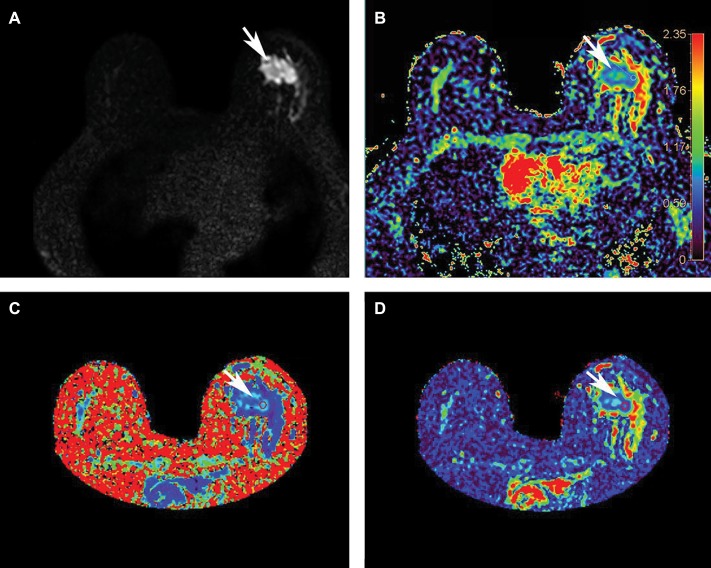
A comparison of diffusion techniques and metrics from scanning a 57-year-old woman with left breast invasive ductal carcinoma (tumor indicated by the arrow) at 3T. (A) The baseline b = 0 image acquired without diffusion gradients; (B) conventional DWI: apparent diffusion coefficient (ADC) map (scale bar 0-2.35 mm^2^/s), arrow indicating tumor ADC value of 1.090 mm^2^/s; (C) diffusion kurtosis imaging: mean kurtosis map (scale bar 0-3 mm^2^/s), arrow indicating tumor mean kurtosis value of 1.154 mm^2^/s; (D) DTI: mean diffusivity map (scale bar 0-2.8 mm^2^/s), arrow indicating tumor mean diffusivity value of 0.808 mm^2^/s. Reprinted with permission from Li et al. (43); ©2018 International Society for Magnetic Resonance in Medicine.
